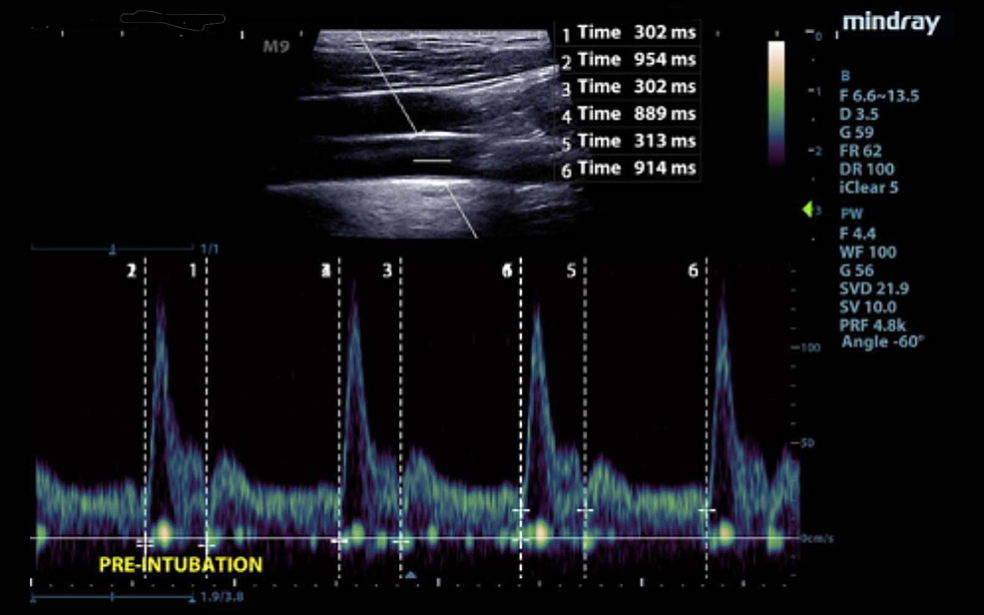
Flow Time and Cycle Time Pulse wave Doppler with measurement of flow time and cycle time over three cycles
